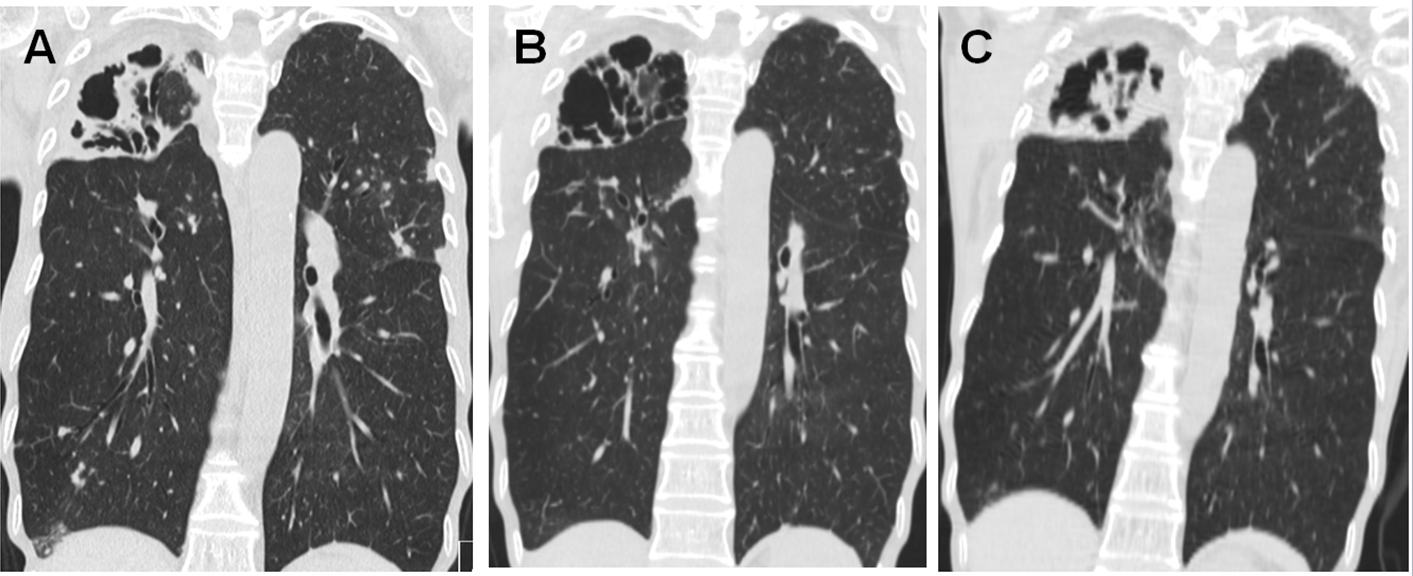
Development of chronic pulmonary aspergillosis in a patient with NTM-LD. Chest computed tomography images are shown for a 69-year-old female patient with *Mycobacterium intracellulare* lung disease. (A) At the time of initiation of antibiotic therapy for *M*. *intracellulare* lung disease, there was a large cavitary consolidation in the right upper lobe. Serum *Aspergillus* precipitin antibody was negative. (B) After 12 months of antibiotic therapy for *M*. *intracellulare* lung disease, cavitary consolidation in the right upper lobe was improved, and sputum cultures for NTM were negative. (C) After 18 months of antibiotic therapy for *M*. *intracellulare* lung disease, the patient’s respiratory symptoms and the consolidation in the right upper lobe were aggravated. Sputum cultures for NTM were negative. However, serum *Aspergillus* precipitin antibody was strongly positive, and chronic pulmonary aspergillosis was diagnosed.
